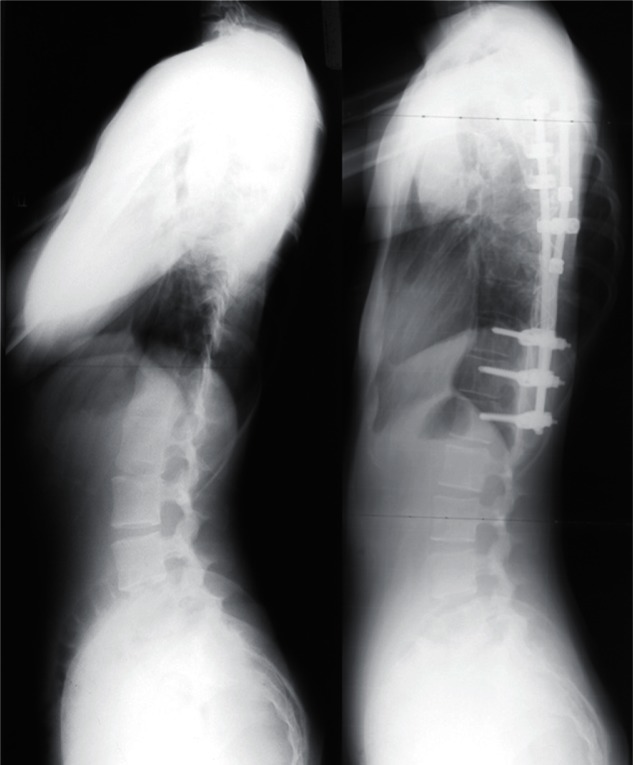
Hybrid constructs using pedicle screws, hooks, and wires. Lateral radiographs
